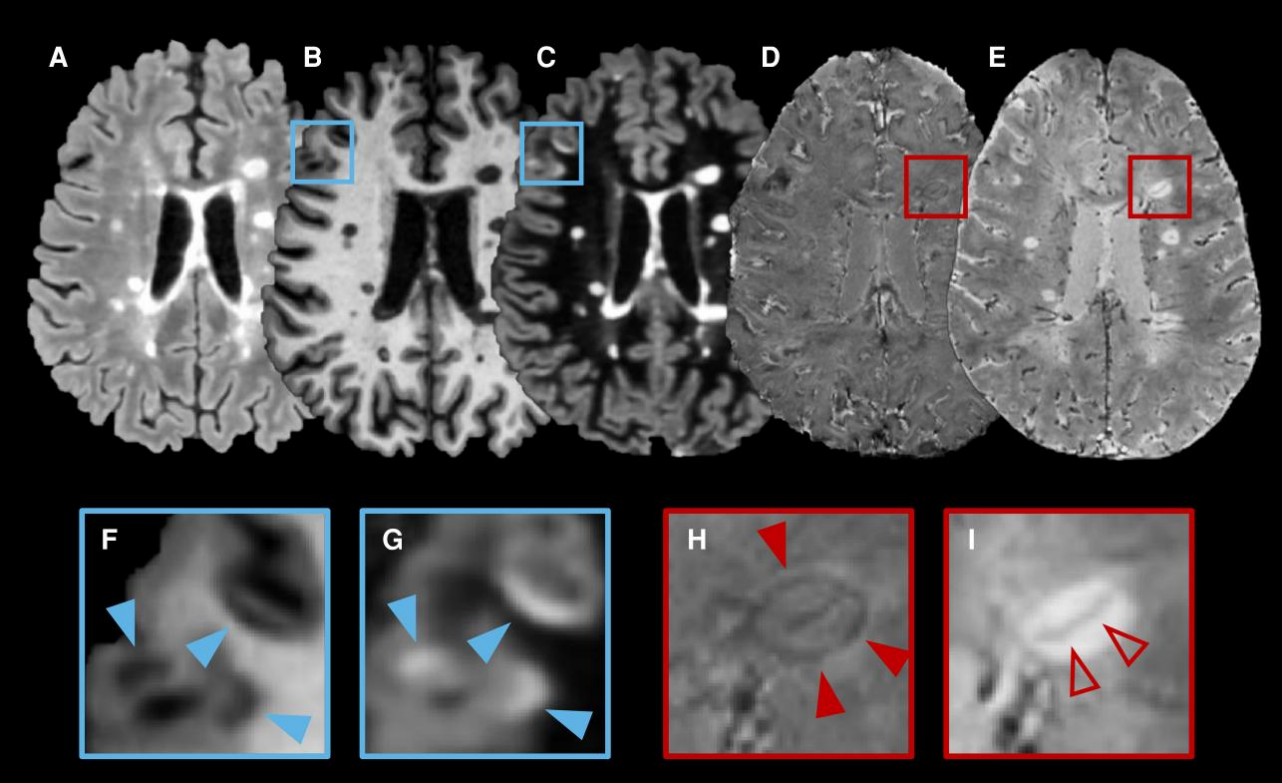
Advanced magnetic resonance imaging (MRI) biomarkers. Representative MRI axial view of a 25-year-old man with relapsing-remitting multiple sclerosis (RRMS) showing the three advanced biomarkers on different contrasts: (A) Fluid Attenuated Inversion Recovery (FLAIR); (B) Magnetization Prepared RApid Gradient Echo (MPRAGE); (C) Double Inversion Recovery (DIR); (D) Echo Planar Imaging (EPI) unwrapped filtered phase; (E) EPI magnitude; (F–G): magnified views of cortical lesions (CL) on MPRAGE (F) and DIR (G) indicated by solid arrows; H: magnified view of a paramagnetic rim lesions (PRL) on EPI unwrapped filtered phase—solid arrows indicating the dark rim; I: magnified view of a lesion showing the central vein sign (CVS) on EPI magnitude—hollow arrow indicating the central vein.
